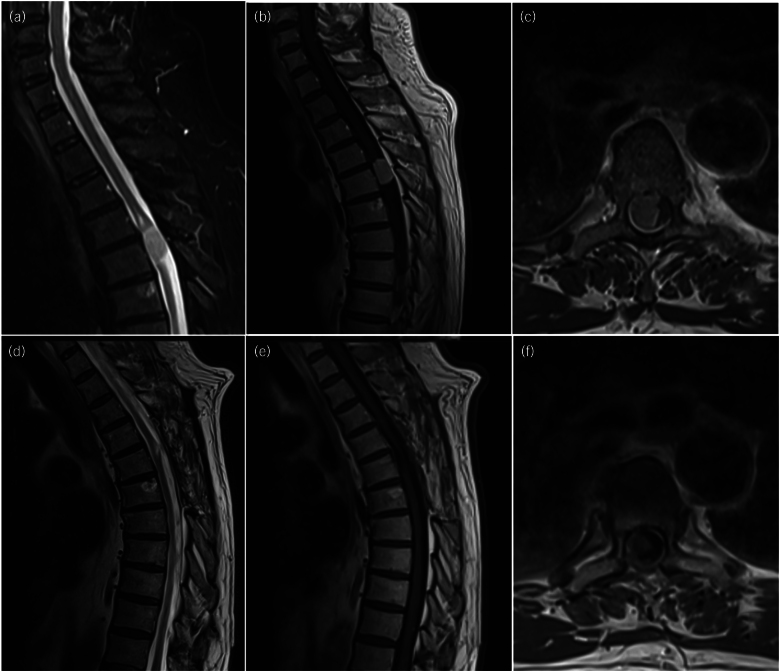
(a–c) Preoperative T2-weighted (a), T1 post-gadolinium (b) sagittal and T1 post-gadolinium axial (c) magnetic resonance imaging (MRI) scans documenting a mid-thoracic meningioma measuring 22×14×11mm in the cranio-caudal, anterior–posterior and latero-lateral axis, respectively. The tumour showed an intermediate T1 and T2 signal with avid homogenous enhancement and a small dural tail at the superoventral aspect. At lesion level there was effacement of the cerebrospinal fluid space and the spinal cord appeared displaced to the left, flattened and distorted, with signal abnormality extending between the levels of T4 and T5, suggestive of myelopathic changes. (d–f) T2-weighted (d) and T1 post-gadolinium (e) sagittal and axial T1 post-gadolinium (f) MRIs one year after surgery, documenting gross total resection of the lesion and absence of recurrence.
